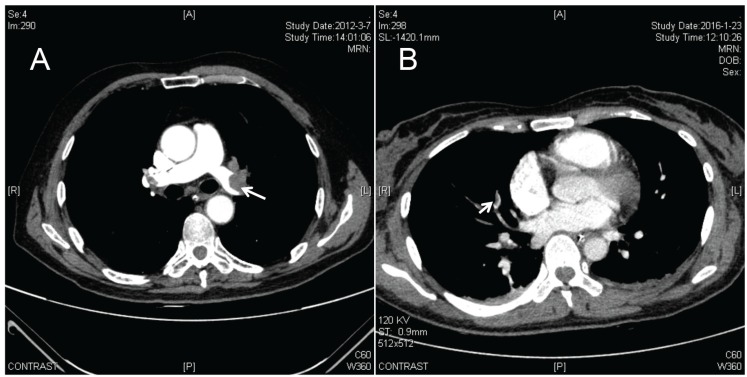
PE diagnosed by CTA of pulmonary artery. (A) Pulmonary artery trunk was embolized. (B) Pulmonary arteriole was embolized. The arrows show the thrombus.
